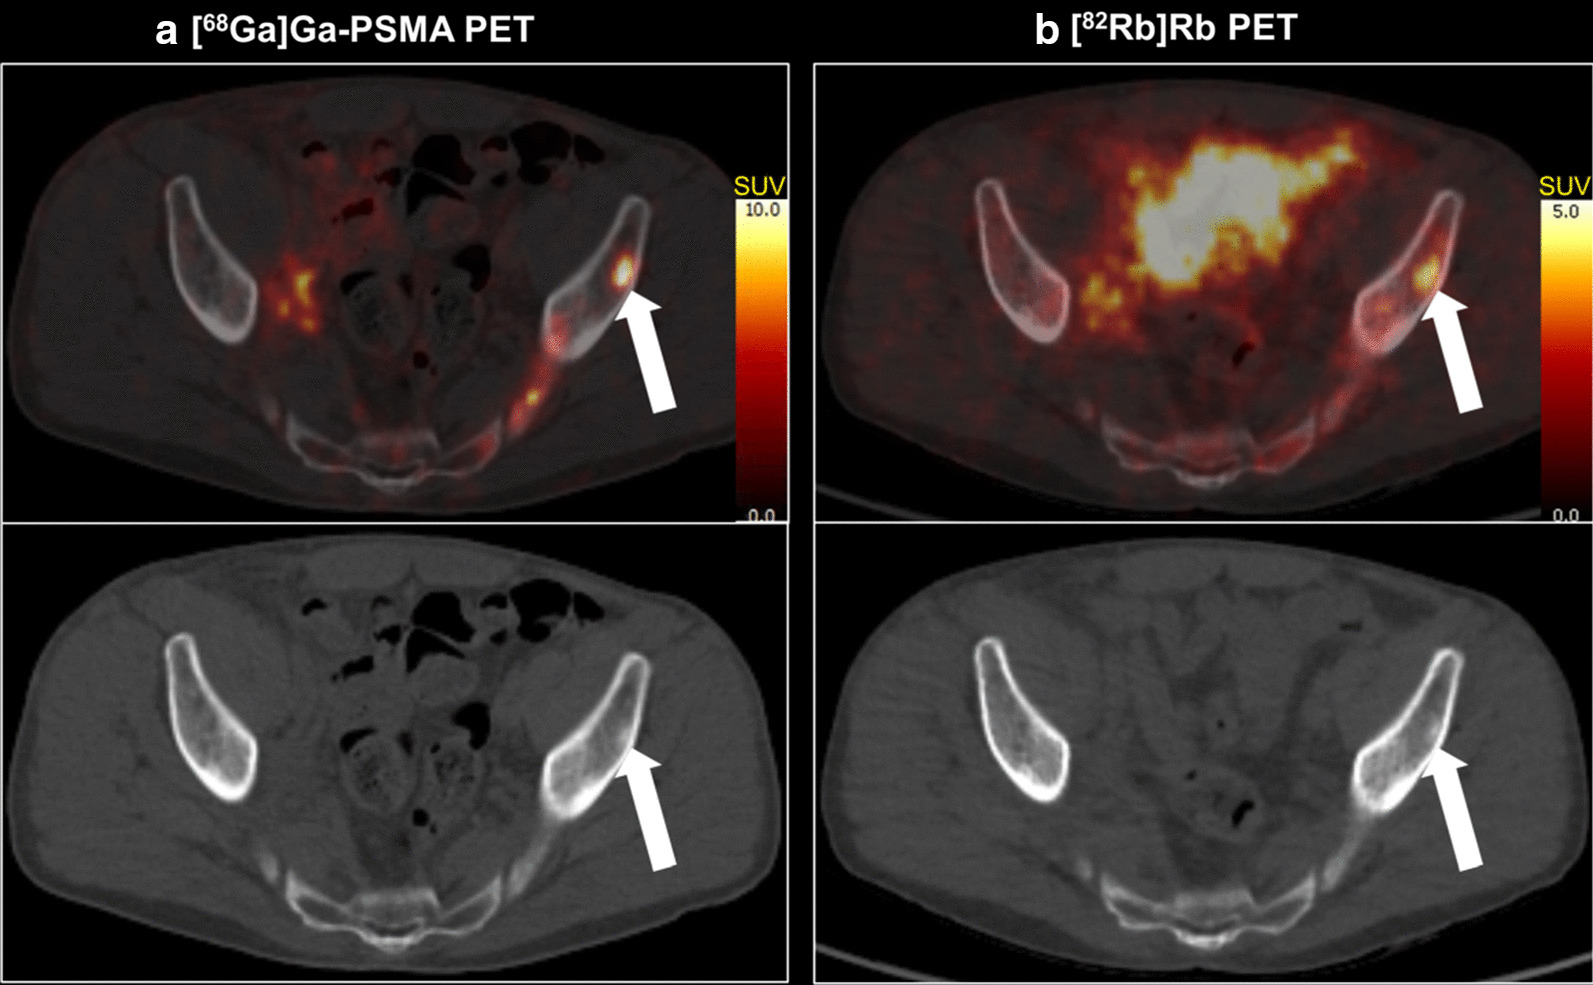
Bone metastasis in the left iliac bone with avid [^68^Ga]Ga-PSMA-11 uptake (a) and highly increased blood flow in the metastatic area (b). The bone window of corresponding low-dose CT reveals slightly enhanced bone density (a, b)
